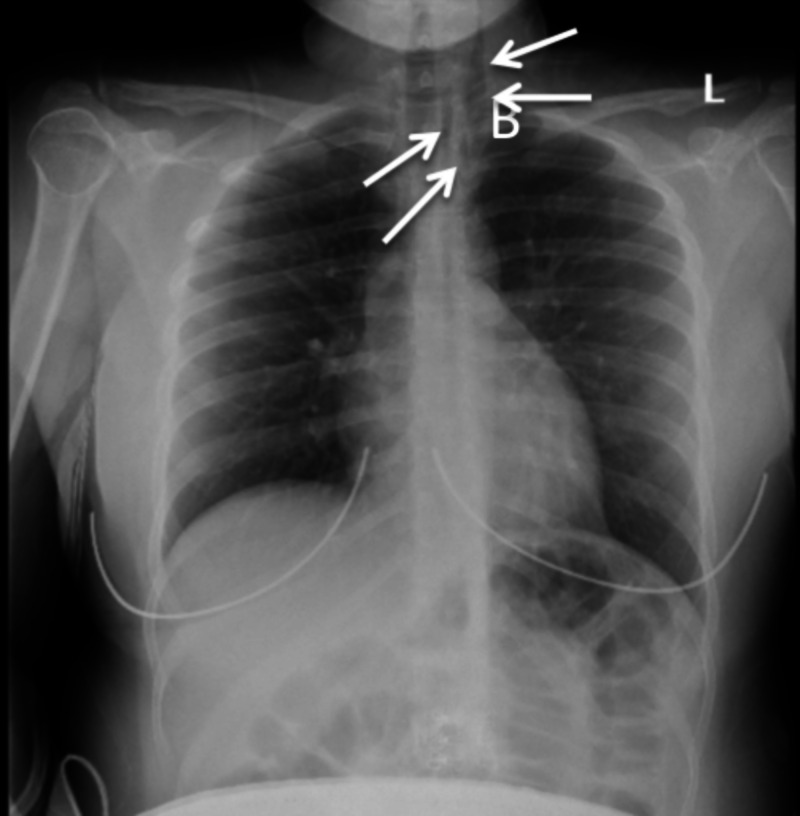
Upright chest X-ray (AP view) on hospital day one The image shows spontaneous pneumomediastinum at the base of the left neck (arrows) AP: anteroposterior
